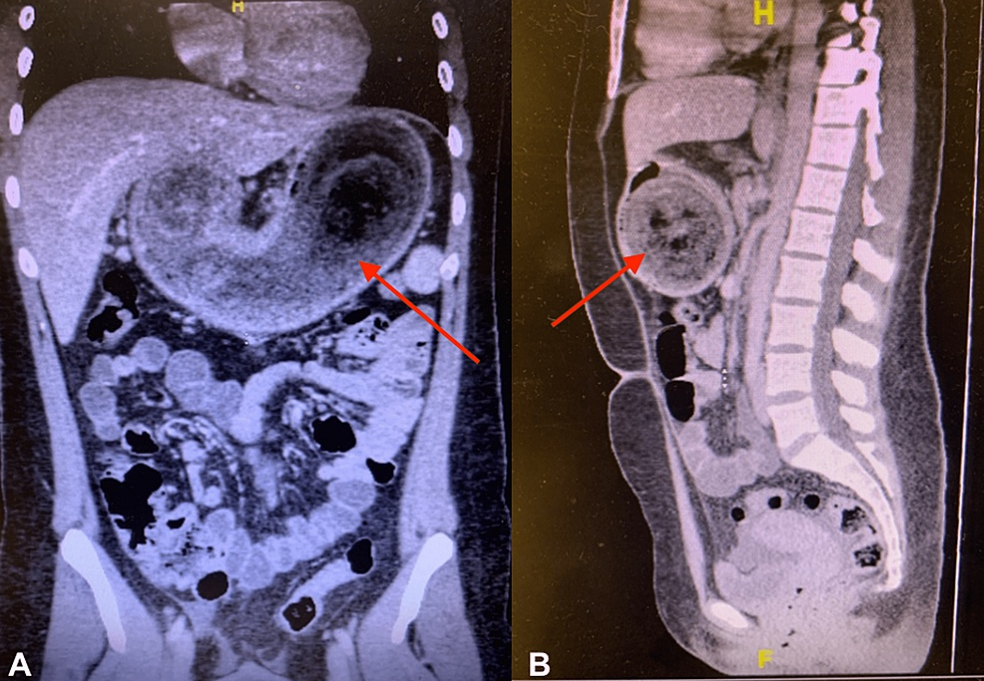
(A) Coronal CT scan showing a large mass in the gastric lumen depicted by the arrow; (B) sagittal CT image showing a large mass in the gastric lumen depicted by the arrow. CT, computed tomography
